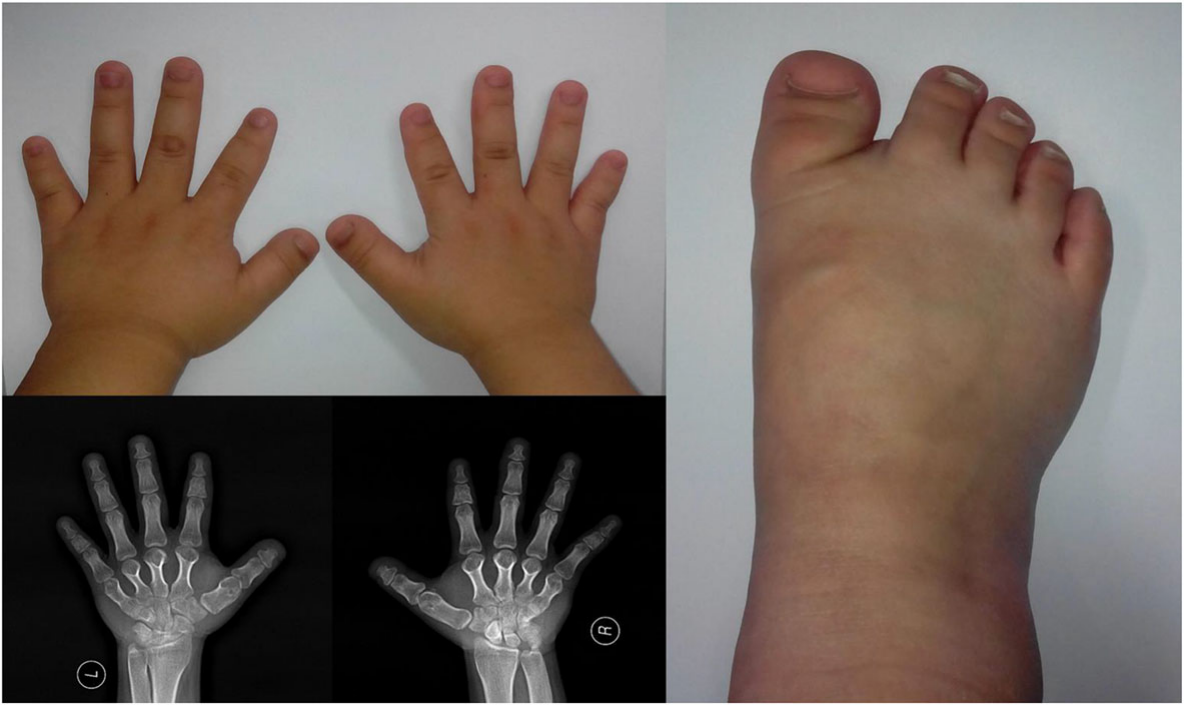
Photos and radiographic images of the patient’s hands and right foot showing short fingers and toes associated with brachydactyly type E (BDE)
